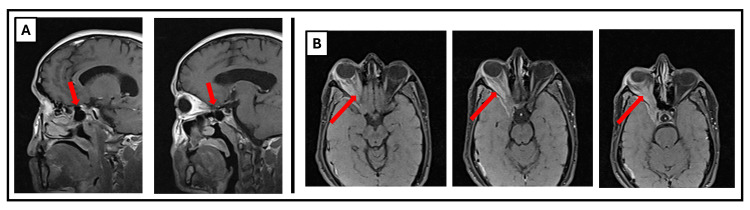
Radiological evaluation of case 2. (A) MRI of the brain sagittal sections with gadolinium showing right para-sellar extra-axial enhancing lesion encasing the right internal carotid artery and extending through the foramen rotundum into the pterygopalatine fossa, inferior orbital fissure, and intraorbital canal. (B) MRI of the brain transverse sections with gadolinium showing the para-sellar lesion extending via the right optic canal and superior orbital fissure to the right orbital apex, including the optic nerve and extraocular muscles, resulting in secondary proptosis.
